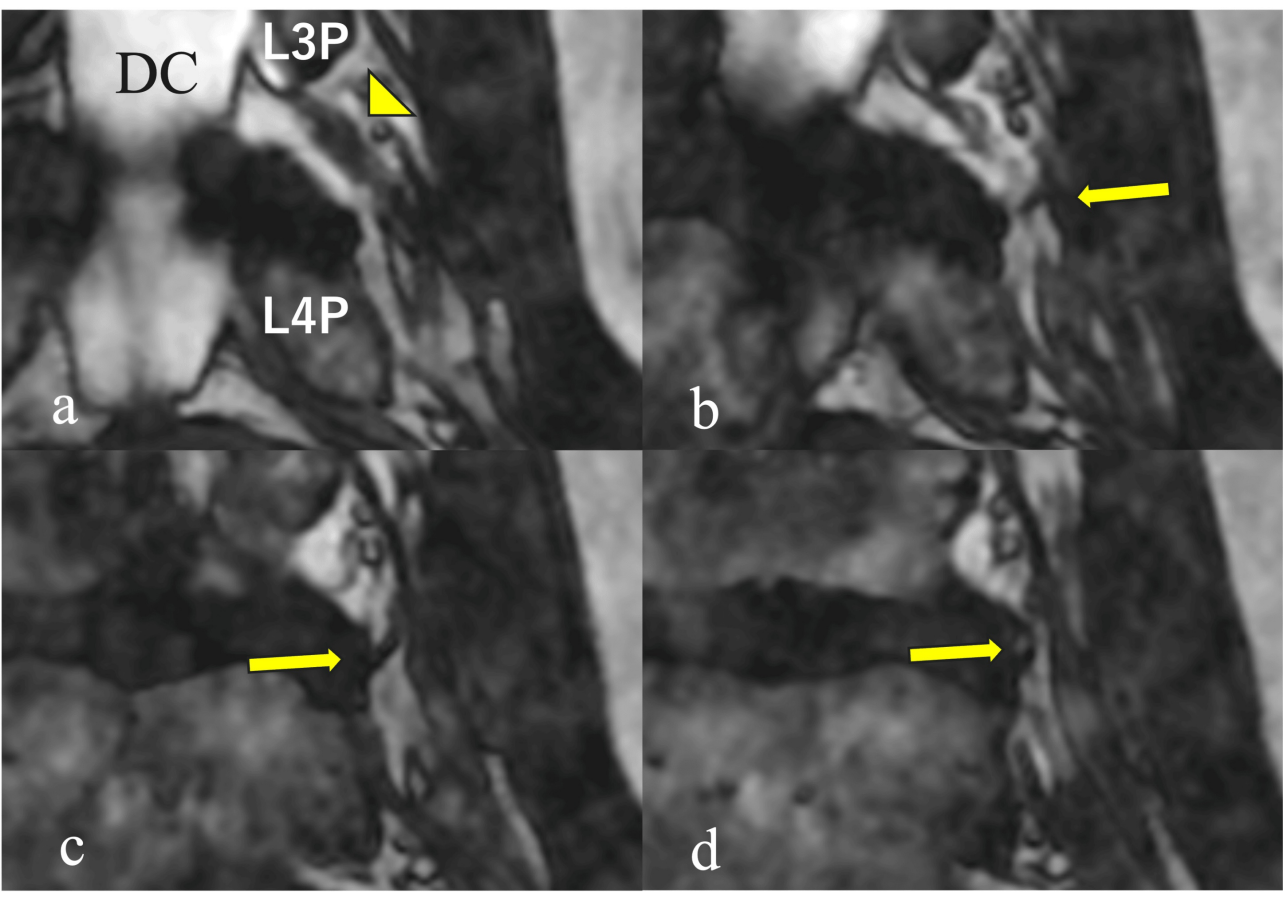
A coronal FIESTA MRI 10 years ago depicting a nerve-like branch arising from the L3 nerve root Coronal FIESTA MRI images obtained before L4–S1 lumbar fusion in case 1 demonstrated a nerve-like structure (arrow) branching from the L3 nerve root (arrowhead) and coursing toward the disc. Panels (a–d) represented sequential coronal slices arranged from dorsal to ventral. (DC: dural canal; L3P: L3 pedicle; L4P: L4 pedicle)
